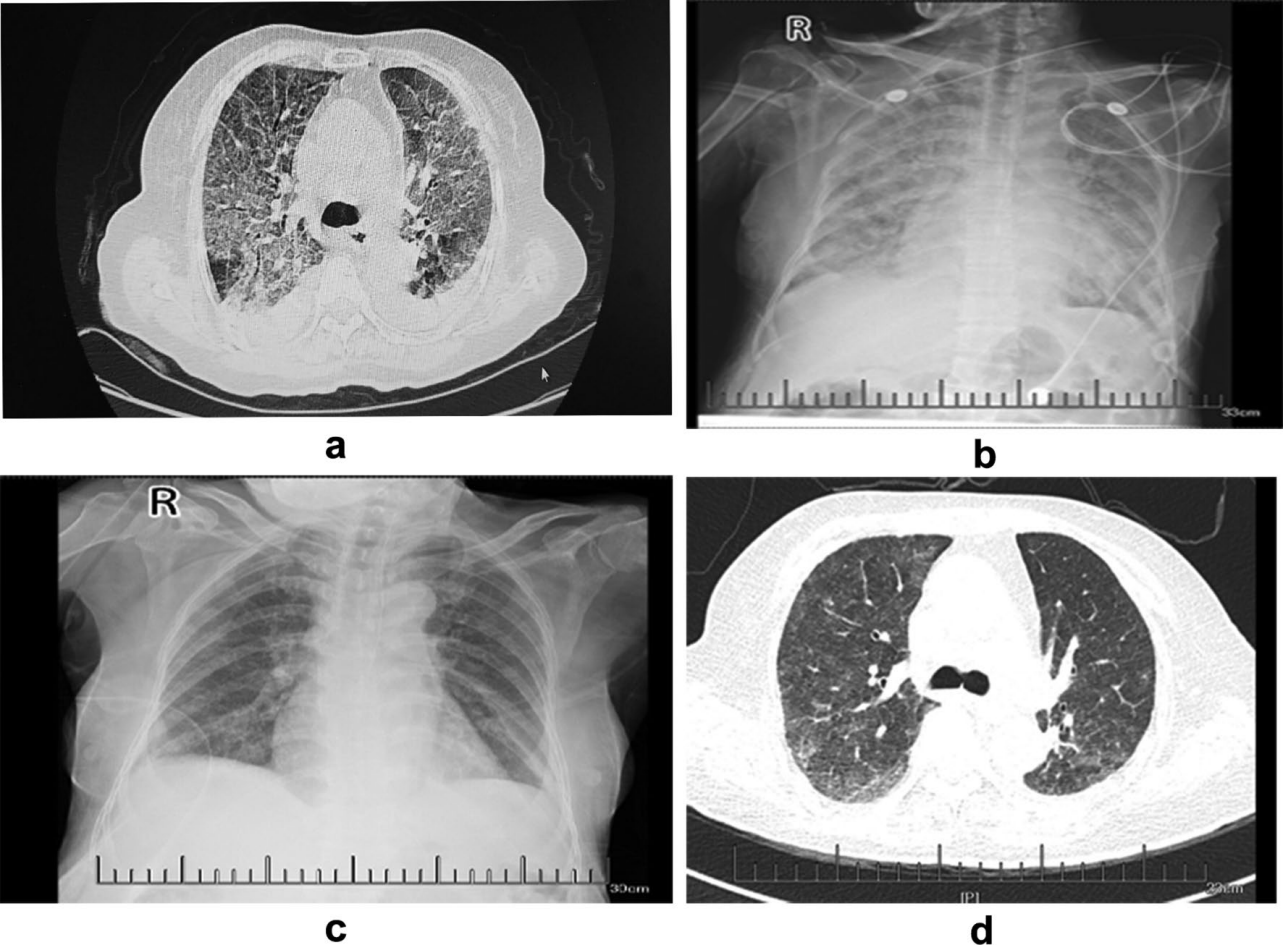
a On the 34th day following crizotinib treatment, the CT showed diffusing bilateral increased ground-glass opacity and reticulation. b Chest X-ray showing diffused bilateral ground-glass opacity. c Chest X-ray showing diffused bilateral ground-glass opacity after treatment with methylprednisolone and bevacizumab. d Chest CT scans showing that the diffuse lesions of both lungs were improved and the pleural effusion decreased significantly.
